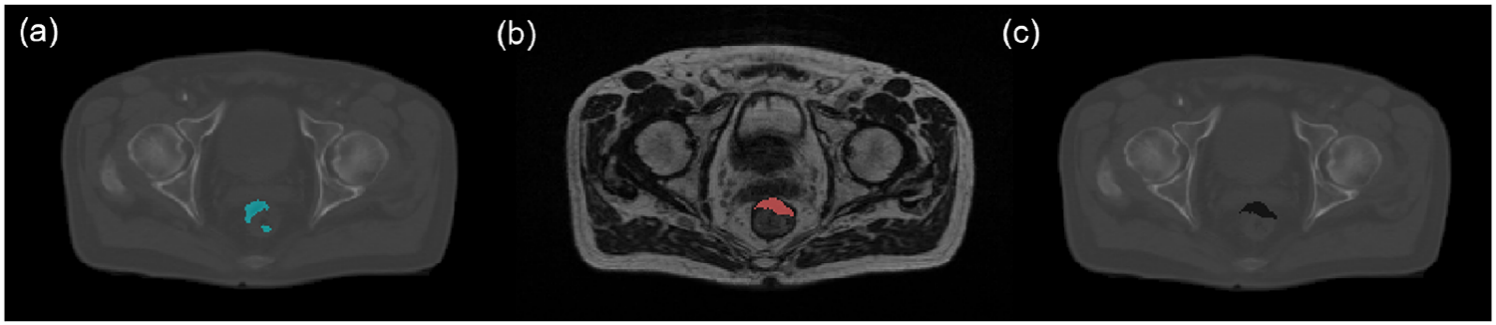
AC correction in the transverse plane of a prostate case: (a) deformed CT with filled AC (blue area), (b) AC contours on MRI (red area indicates the AC contour), (c) corrected CT. AC, air cavitie; CT, computed tomography; MRI, magnetic resonance imaging.
